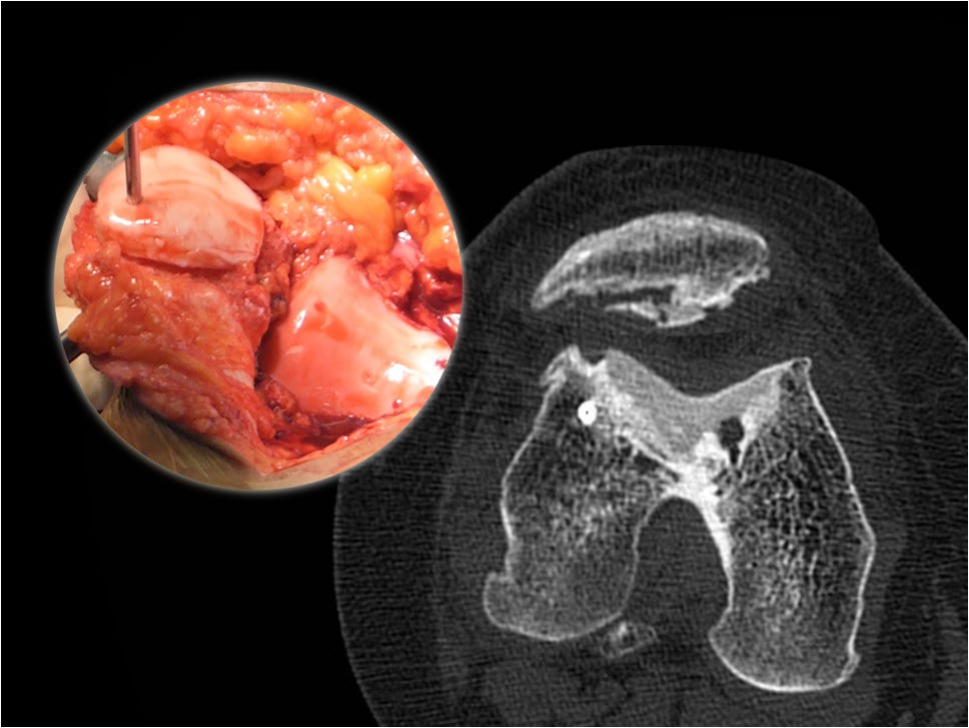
CT scan taken at 24 months and surgical image of a trochlear and patellar FOCA obtaining a high ACTOCA score (6 points)
